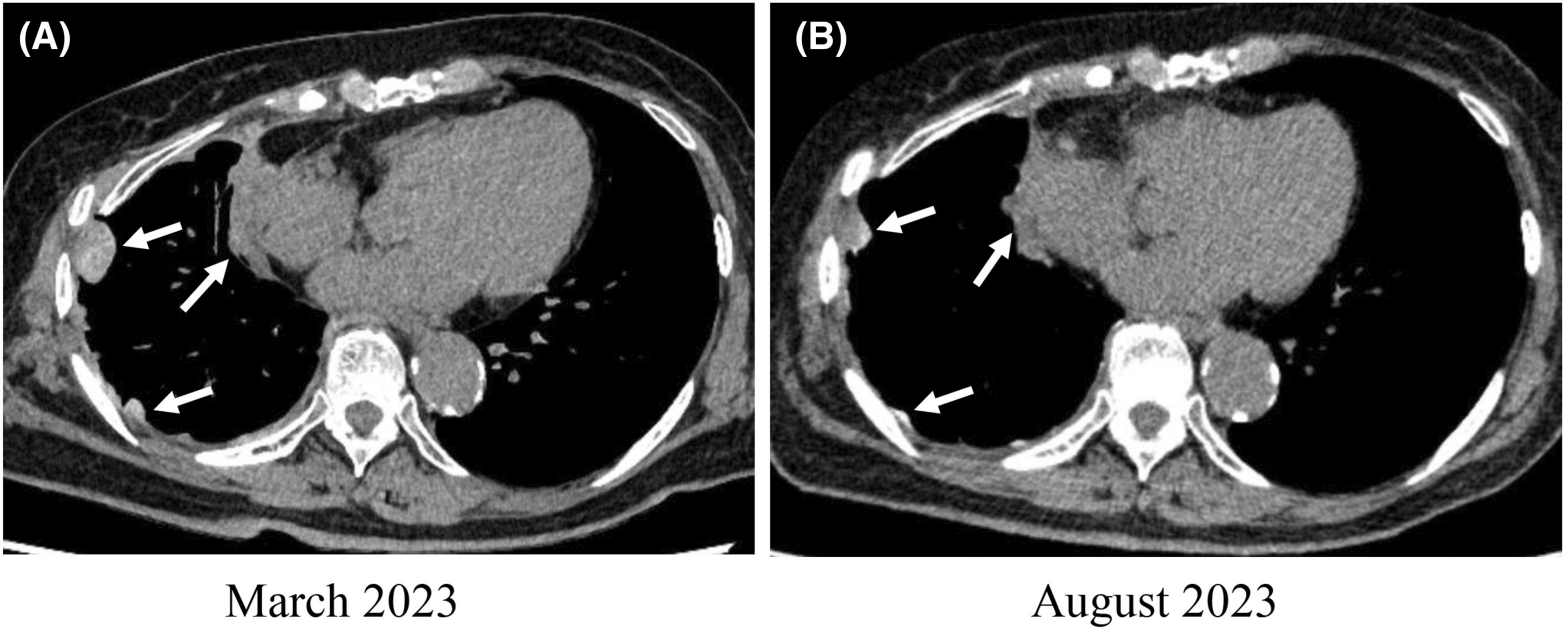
Chest CT taken before (A) and after (B) five courses of pembrolizumab administration. A partial response for pleural recurrence (arrows) was achieved.
